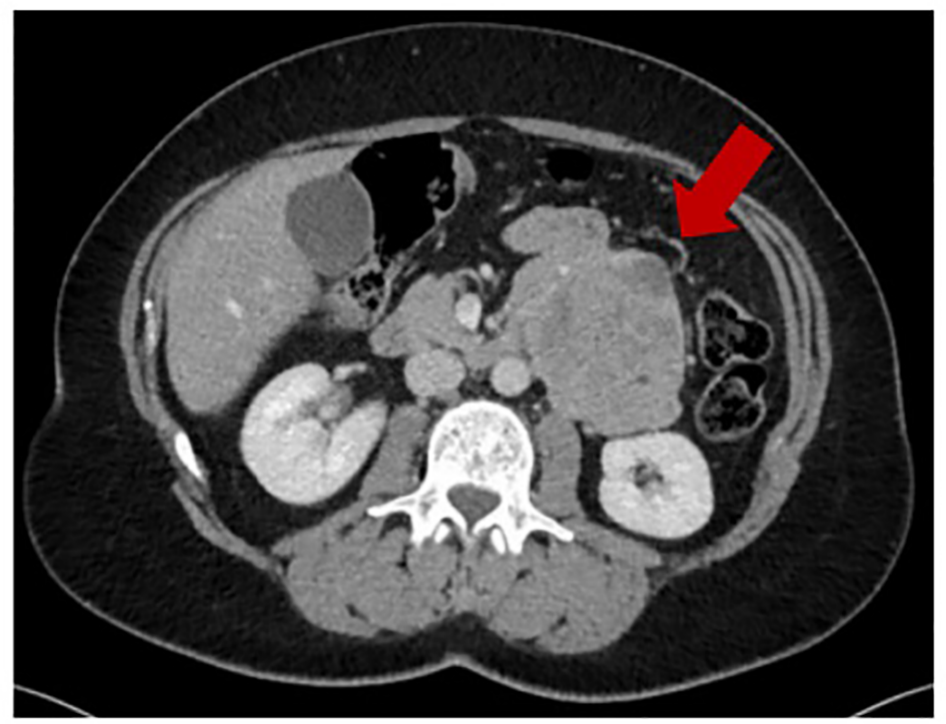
Baseline CT scan (16/December/2016).
